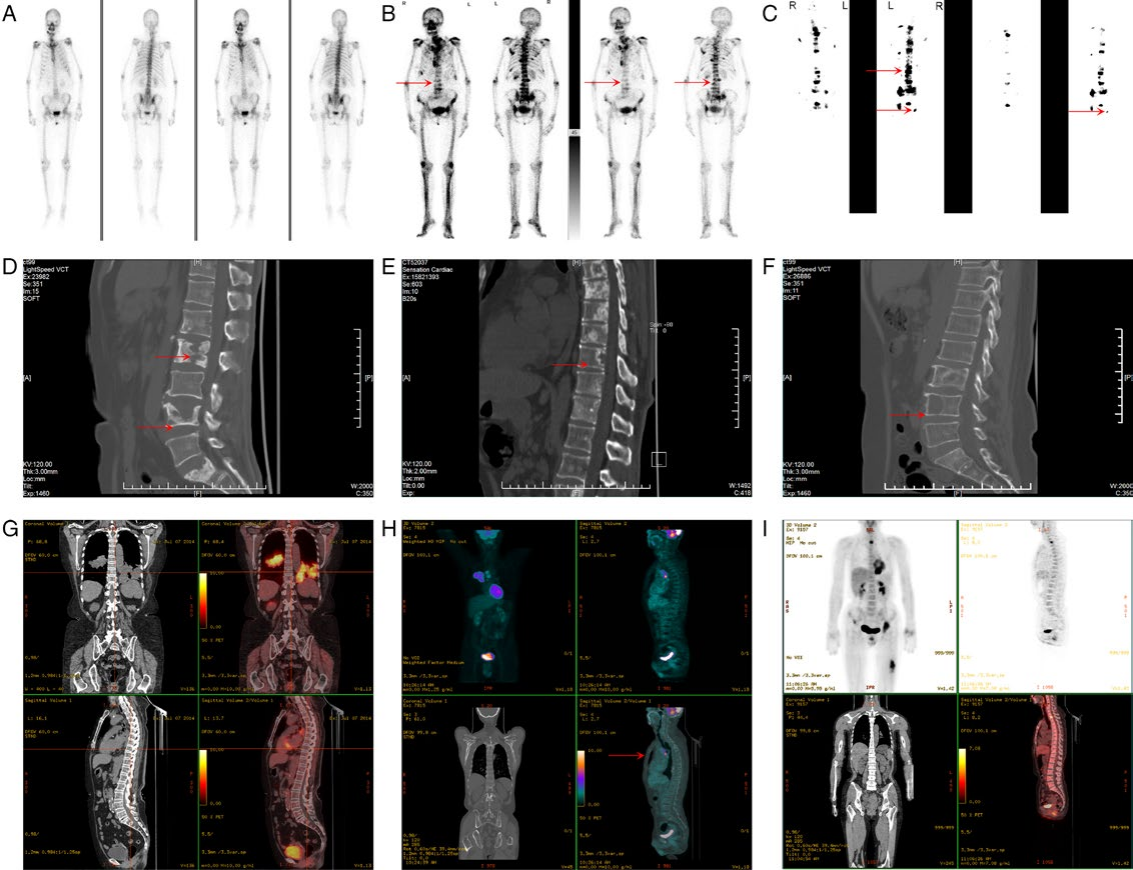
Imaging features of LUAD with BM. (A) ECT from LUAD without BM. (B–C) ECT from LUAD with BM. (D‐F) CT from LUAD with BM. (G‐H) PET‐CT from LUAD without BM. (I) PET‐CT from LUAD with BM. Arrows indicate the focus of BM (arrows).
